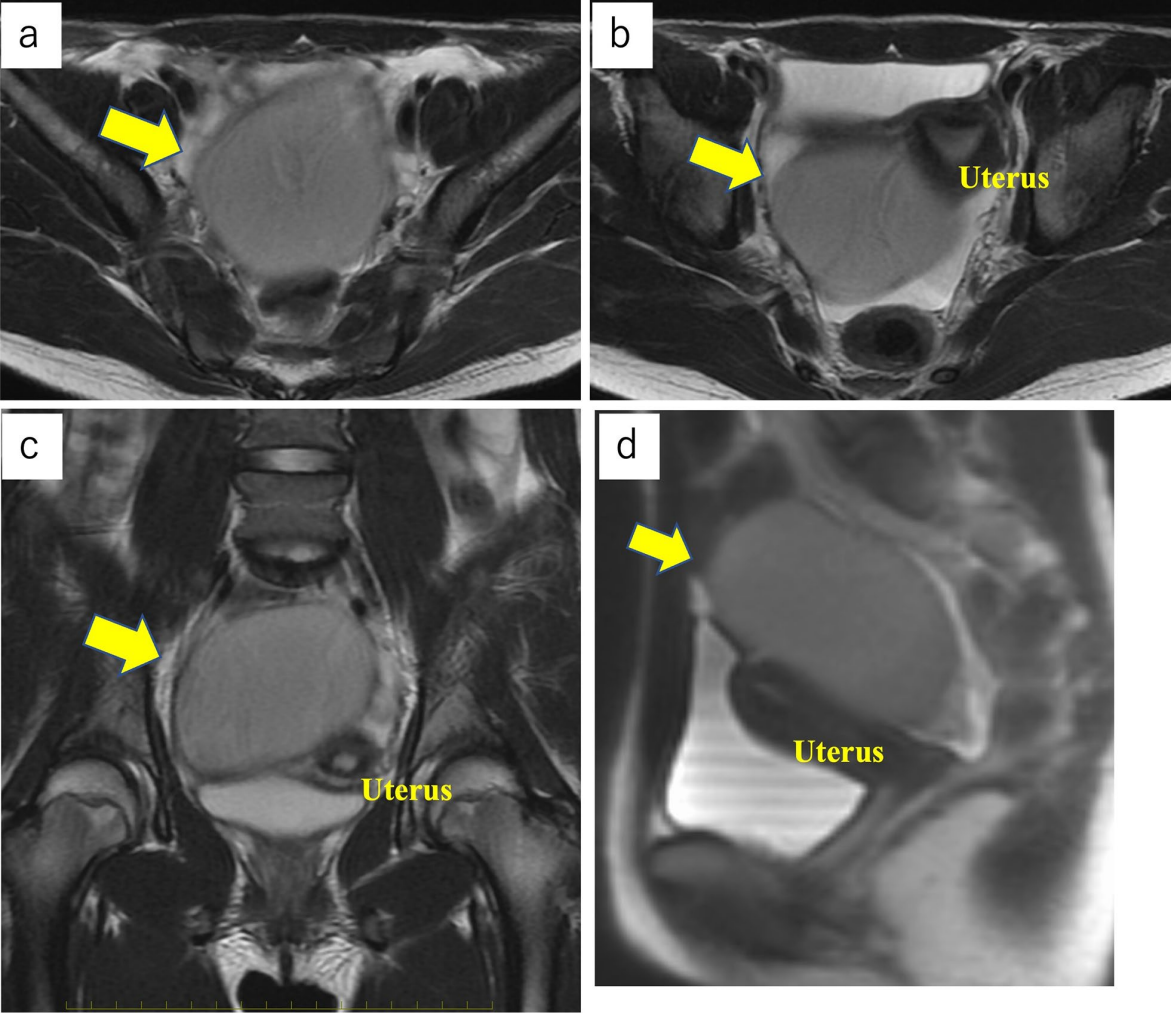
Preoperative pelvic magnetic resonance imaging. Preoperative images show a tumor (yellow arrow) that was a cystic mass measuring 90 × 65 mm with a smooth margin and homogeneous signal intensity on the right dorsal side of the uterus (a–d). Coronal image (c), sagittal image (d). T1-weighted images present slightly higher signals than those of water, and T2-weighted images show low signals. No apparent communication was found between the cyst and the intestinal tract
